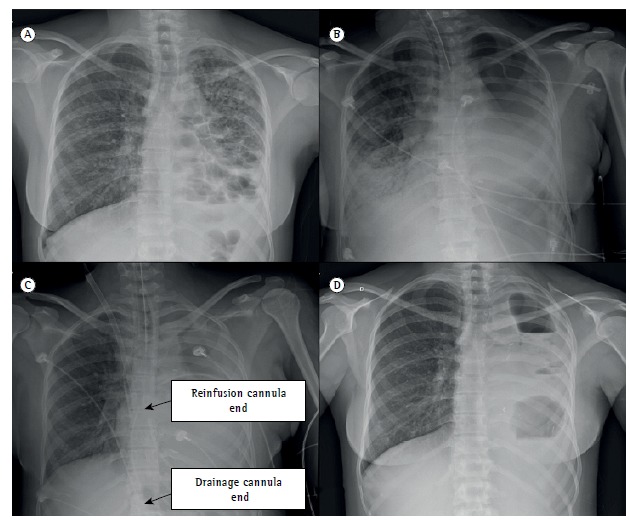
Chest X-rays showing the progression of the patient. In A, chest X-ray
